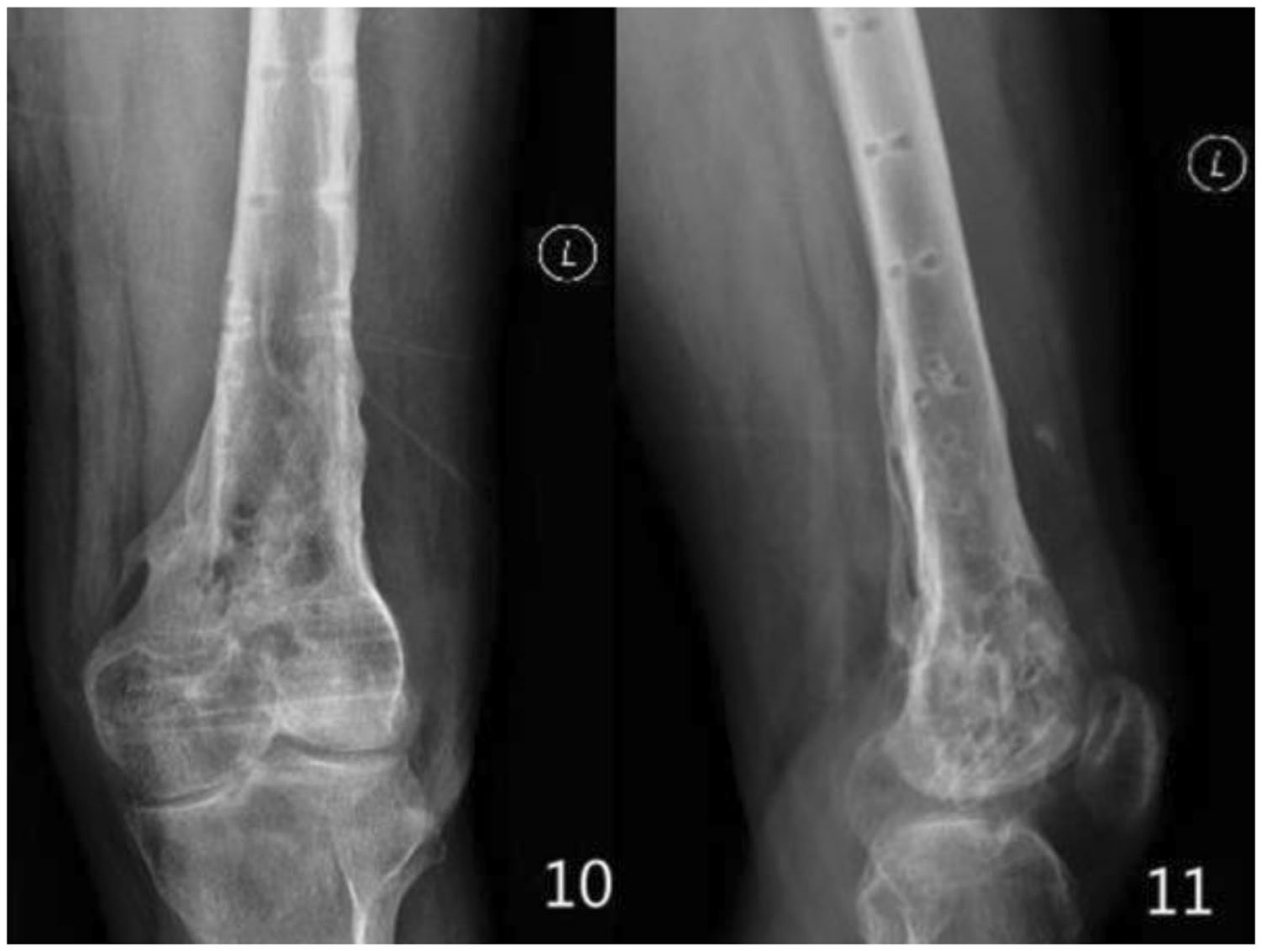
10, 11 the X-ray of the femur, taken after the plates were taken out.
